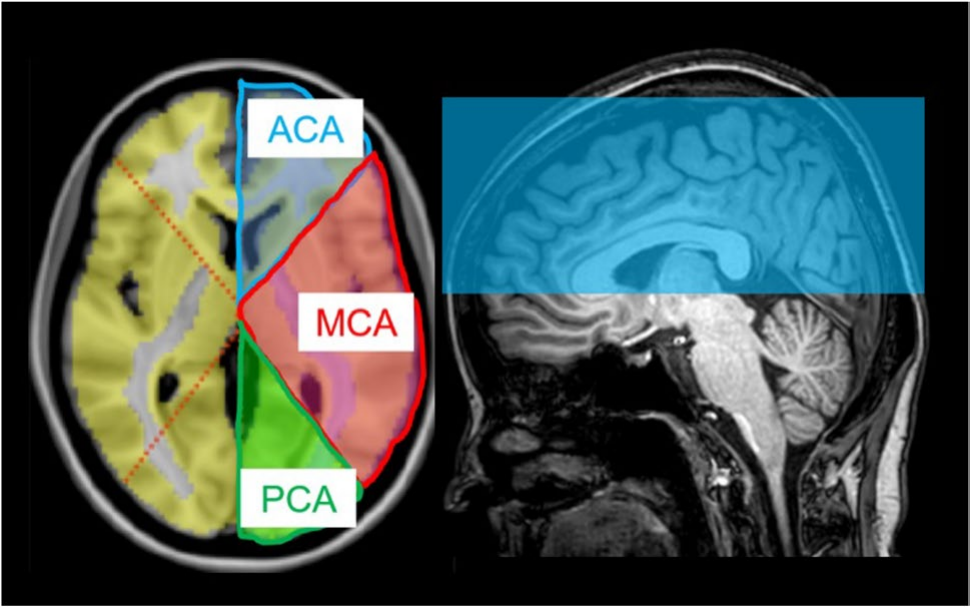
The regions of interest based on the Alberta Stroke Program Early CT Score (ASPECTS). The regions of interest are placed in the anterior cerebral arterial (ACA, blue), middle cerebral arterial (MCA, red), and posterior cerebral arterial (PCA, green) territories, roughly corresponding to the Alberta Stroke Program Early CT Score (ASPECTS) based on the Montreal Neurological Institute brain template. Each cerebral arterial territory is measured from the basal ganglia level to the vertex (right: blue area).
